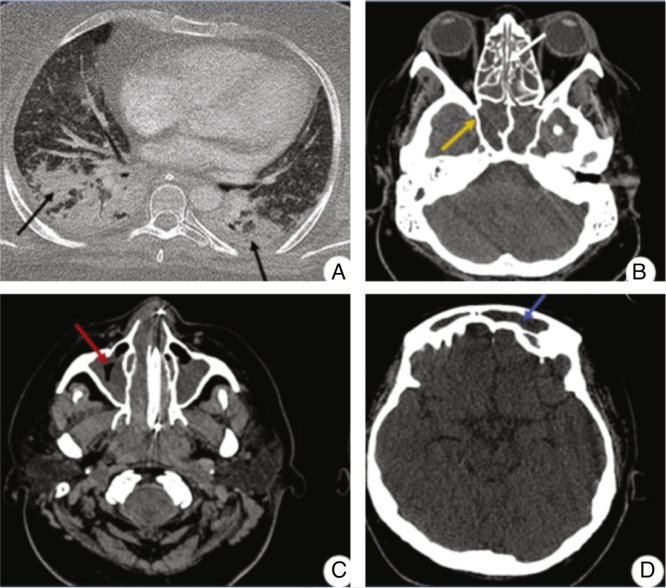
A, Chest tomography showing a pattern of parenchymal consolidation with intermingled air bronchograms, notably in the posterior region of the pulmonary fields (black arrows). (B, C, and D) CT scan of the sinuses showing diffuse veiling of the paranasal sinuses, characterized by material with soft tissue density filling the maxillary (red arrow), sphenoid (yellow arrow), and frontal sinuses (blue arrow), and the ethmoidal cells (white arrow).
